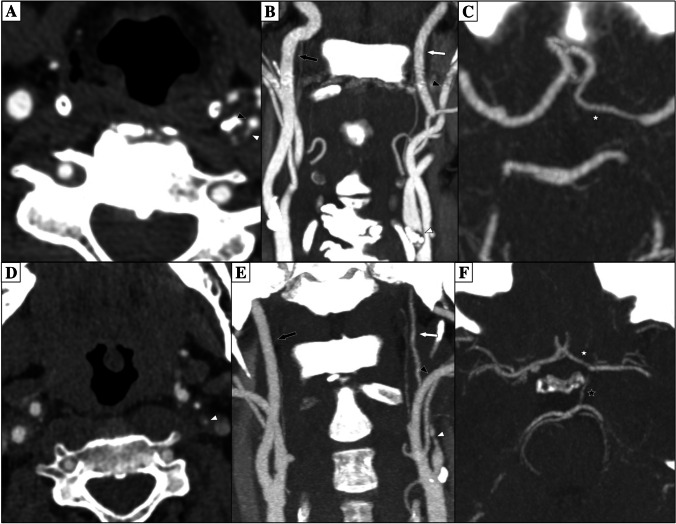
CTA of two examples of left-sided near-occlusion. (A-C) Near-occlusion without full collapse. (A-B) After a severe stenosis, the distal ICA is normal-appearing but smaller than the contralateral ICA and approaching the size of ipsilateral external carotid artery. Parts of the distal left ICA not in the plane. (C) Left A1 smaller than right, Pcom not seen. On PC-MRI, ICA flows were 312 ml/min right and 72 ml/min left; A1 flows were 185 ml/min right and -42 ml/min (reversed) left; Pcom and OA not seen bilaterally. (D-F) Near-occlusion with full collapse. (D-E) After a severe stenosis, the distal left ICA is threadlike, clearly smaller than the contralateral ICA and ipsilateral external carotid artery. (F) Virtually similar A1s and left Pcom seen. On PC-MRI, ICA flows were 264 ml/min right and seen as weak antegrade signal left (not assessable by the algorithm, approximated to 10 ml/min); A1 flows were 198 ml/min right and -61 ml/min (reversed) left; Pcom left -52 ml/min (towards the MCA), right not seen; OA right antegrade, left not seen. In all figures: White arrowhead is stenosis; White arrow is ipsilateral distal ICA; Black arrowhead is ipsilateral external carotid artery; Black arrow is contralateral ICA; White star is ipsilateral A1; Black star is probable ipsilateral Pcom;
